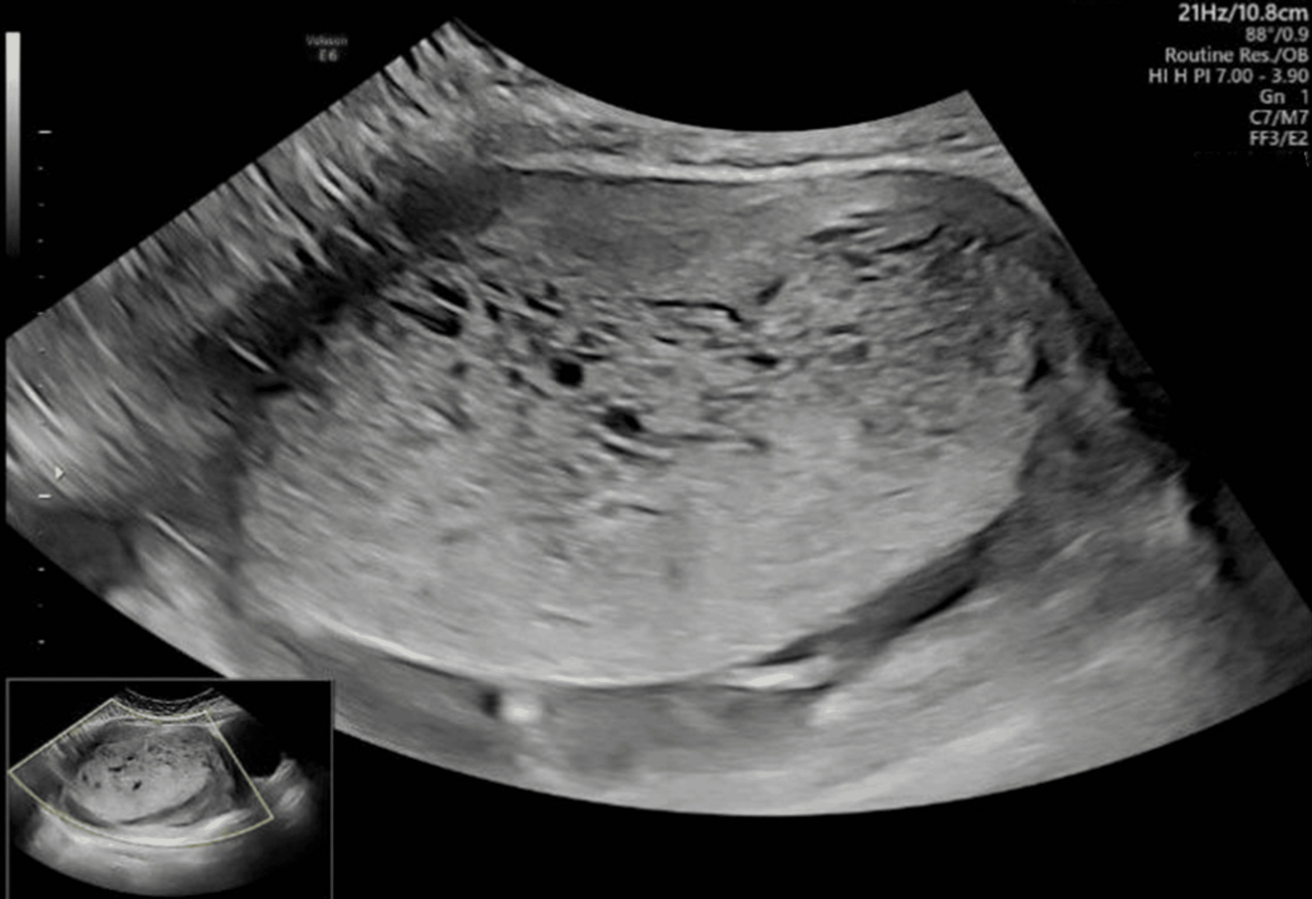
Ultrasound image demonstrating the abnormal gestational sac with features of complete molar degeneration, including diffuse cystic changes and absence of vascularity on Doppler imaging.
